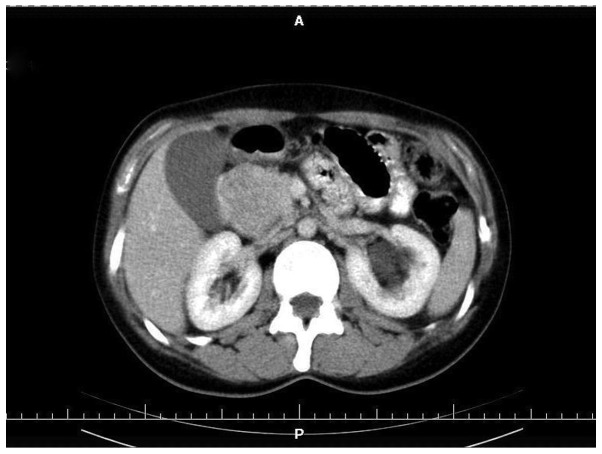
Tumor of the head of the pancreas on computed tomography scan.
